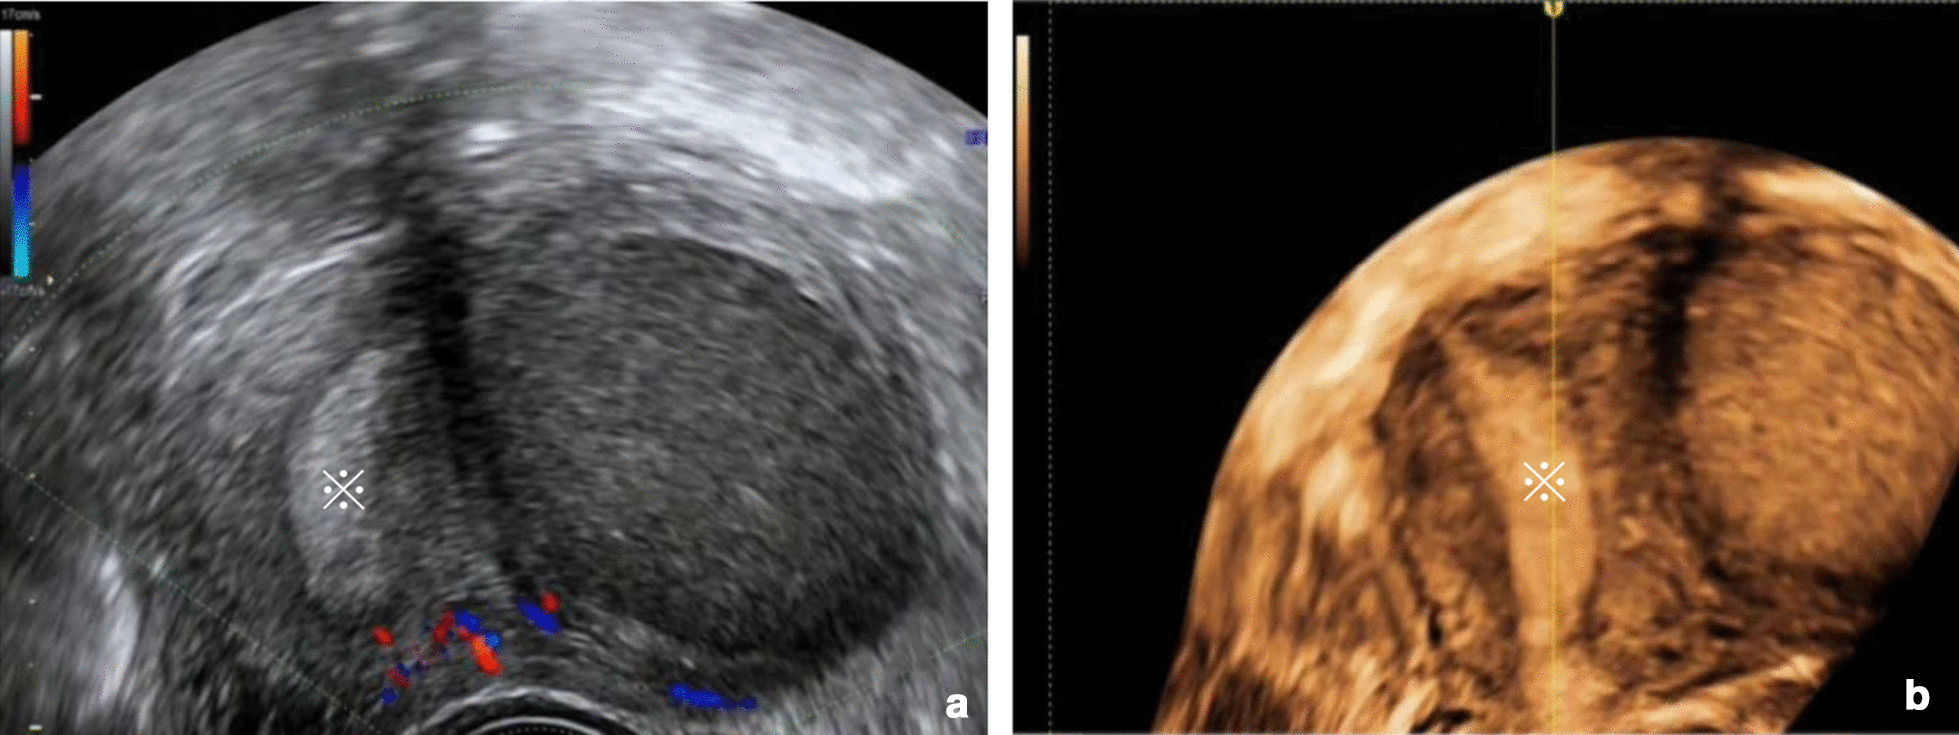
Three dimensional ultrasound images. a The uterus showing an normal shape of uterine cavity. b A well-circumscribed cystic lesion of 4.5 × 4 × 5.0 cm in the left anterior wall, and well separated from the normal uterine cavity
